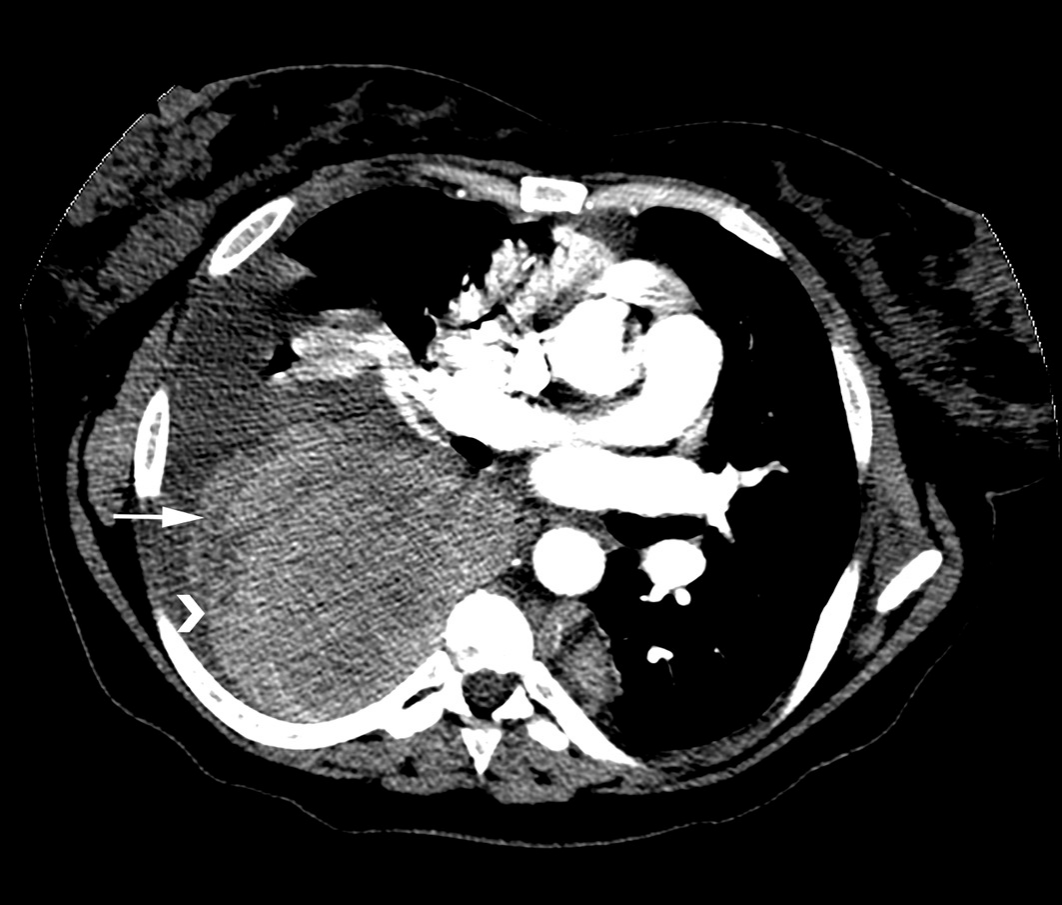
CT pulmonary angiogram at presentation showed layering haemothorax (arrow) adjacent to a large extramedullary haematopoietic mass (arrowhead) within the posterior mediastinum.
